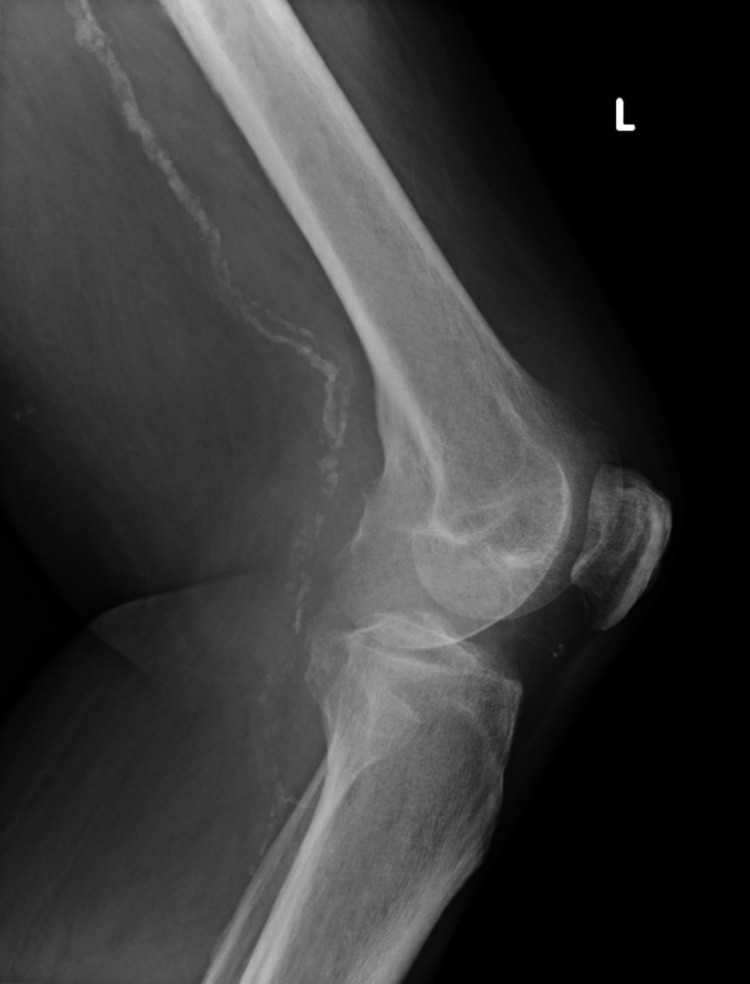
X-ray left knee (Lateral view)
